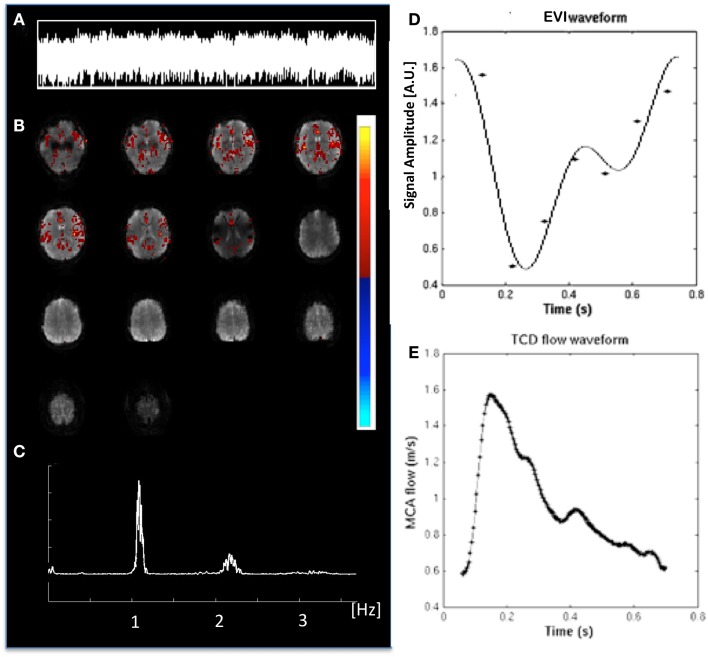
Cardiac-related signal pulsation measured in a healthy control using MEVI2 (TR: 136 ms). (A) ICA time course of the pulsation. (B) ICA spatial map shows pulsation predominantly in insular cortex and medial gray matter (*z*_max_ = 12.5). The color scale for ICA is shown on the right. (C) Corresponding raw power spectrum shows the cardiac frequency and its first harmonic. (D) Fitted MEVI2 signal time course, which is inverted with respect to (E) a typical Transcranial Doppler Ultrasound waveform from the middle cerebral artery (from Wagshul et al., 2011).
